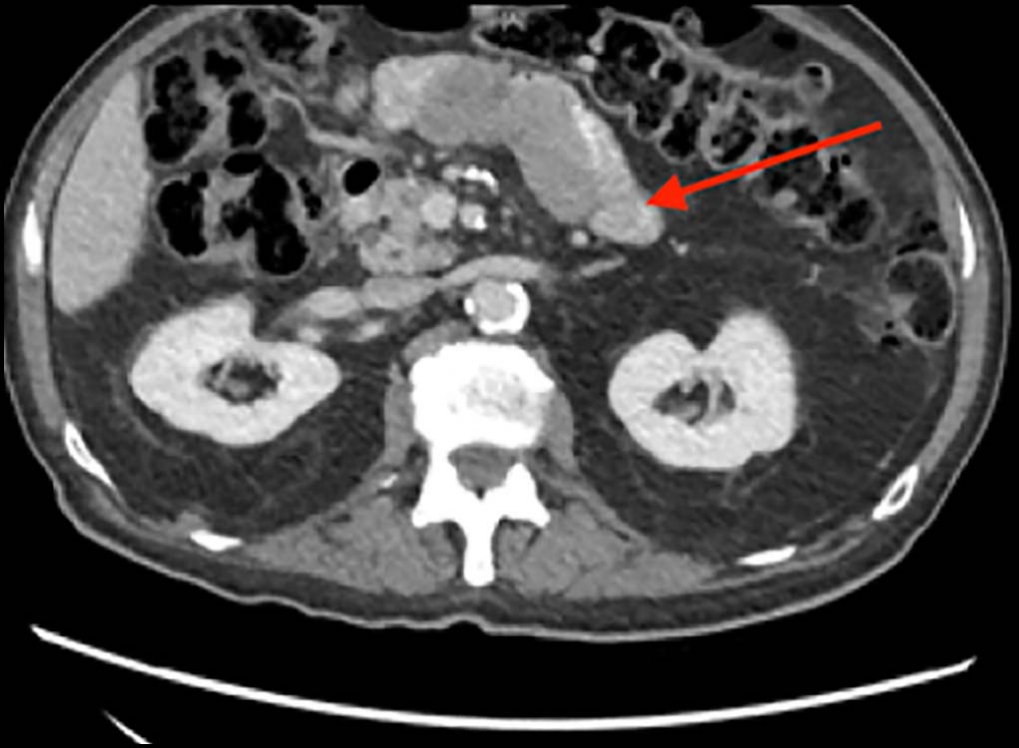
Abdominal and pelvic computed tomography imaging displaying a lymph node inseparable from a loop of proximal small bowel measuring 7.3 × 2.4 cm.
